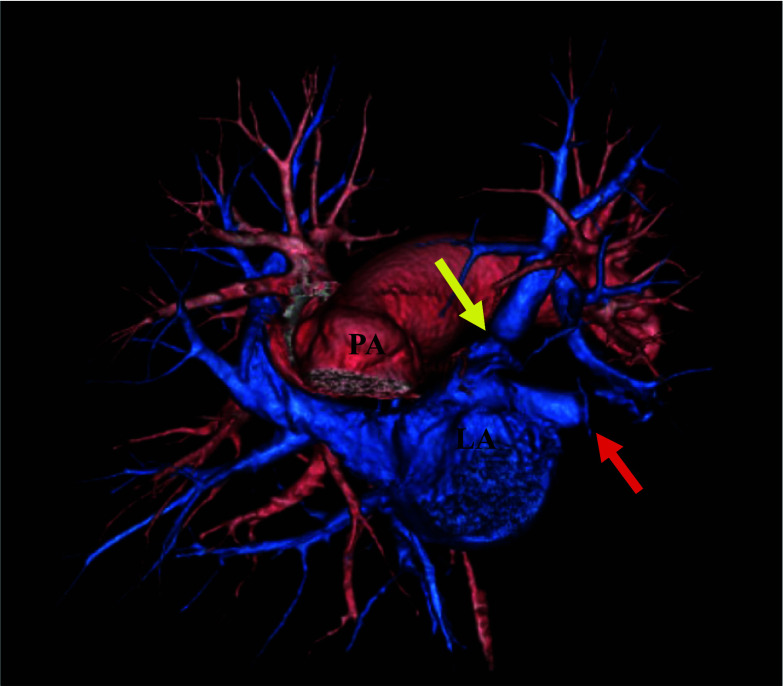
Postoperative contrast-enhanced computed tomography. The anastomotic area was intact and patent (yellow arrow). The red arrow indicates the stump of the left lower pulmonary vein
